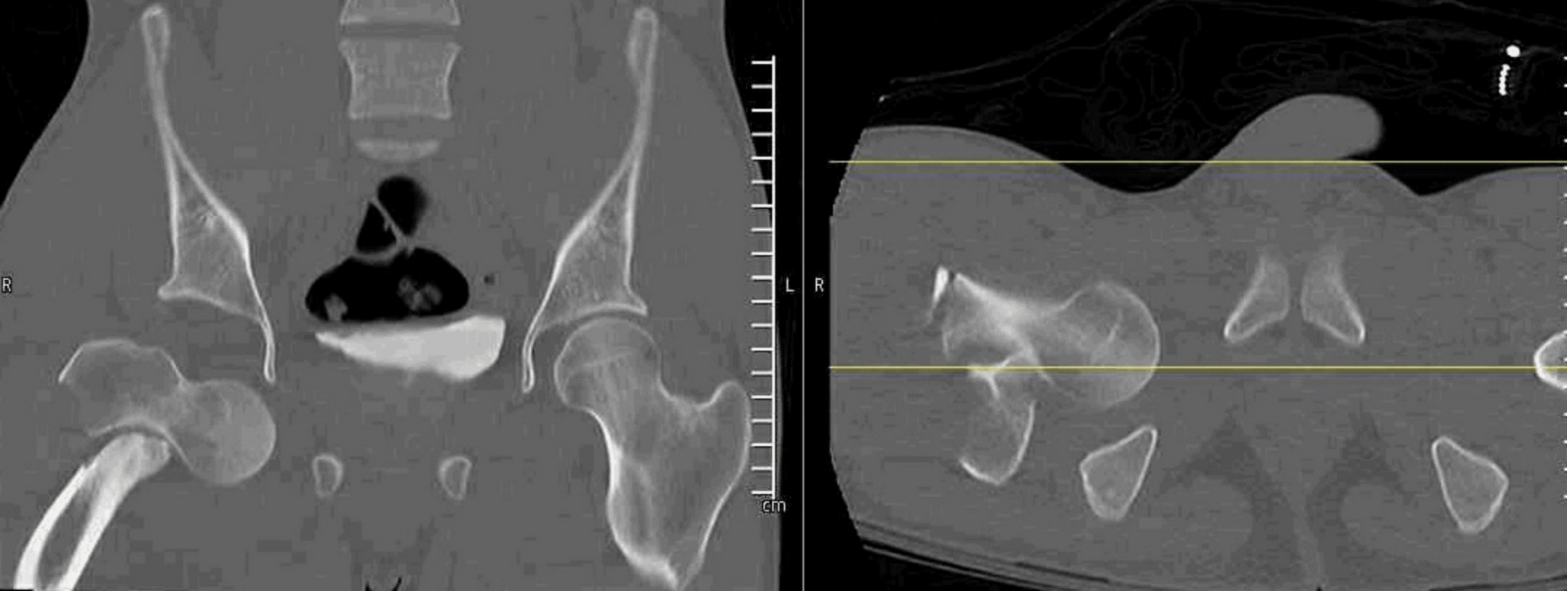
Coronal and corresponding axial CT images showing an ipsilateral intertrochanteric hip fracture associated with a direct inferior hip dislocation.
